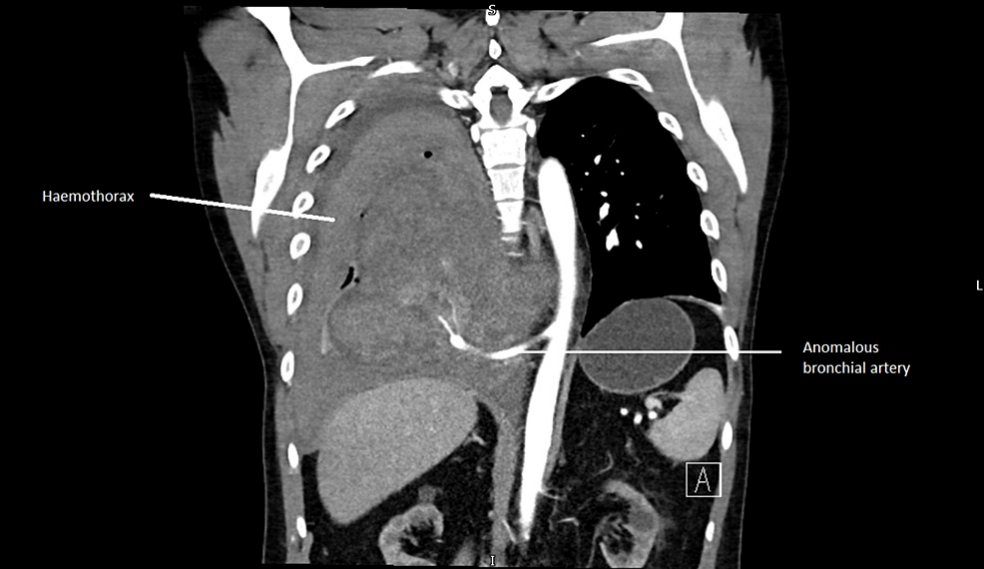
CT chest coronal image, scan done after eight days of admission showing right-sided haemothorax from anomalous bronchial artery bleed
